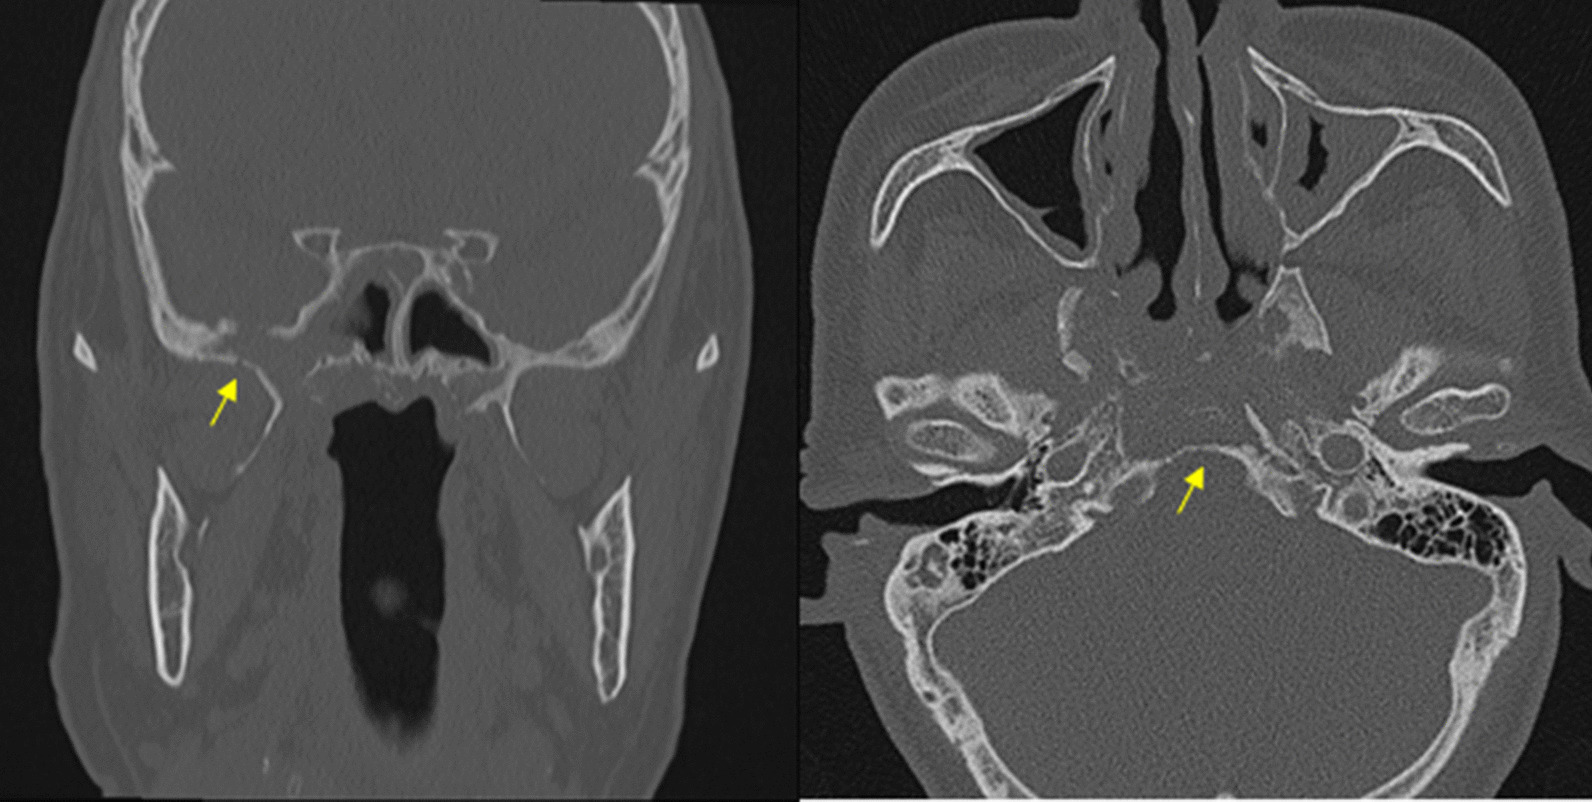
4 Months follow-up CT showing improved osteolytic change
